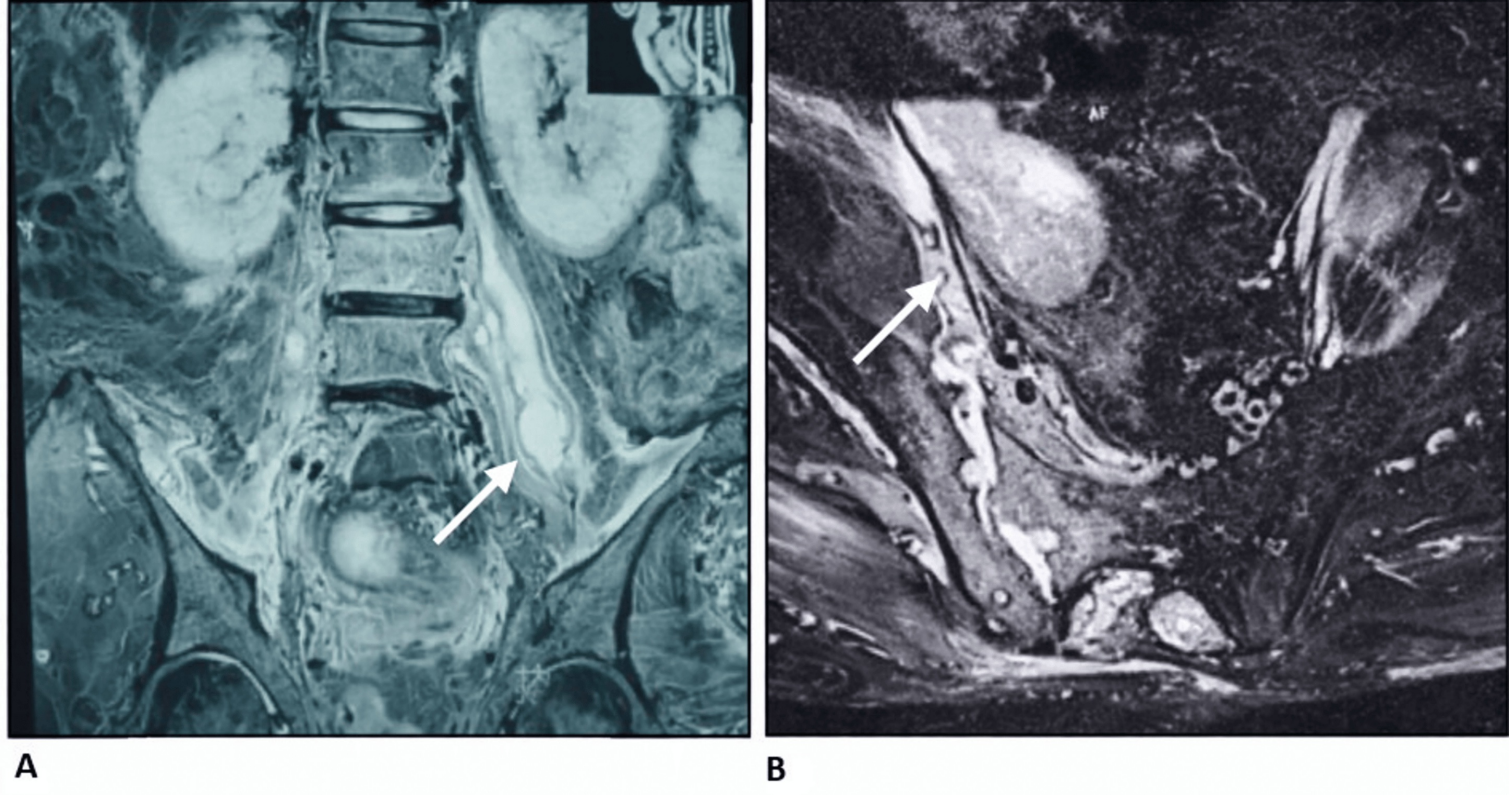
Magnetic resonance imaging of the sacroiliac region demonstrating sacroiliitis with associated psoas collection. (A) Coronal magnetic resonance image demonstrating an associated psoas collection consistent with abscess formation (arrow). (B) Axial magnetic resonance image demonstrating sacroiliac joint involvement with joint space irregularity and adjacent inflammatory change (arrow).
